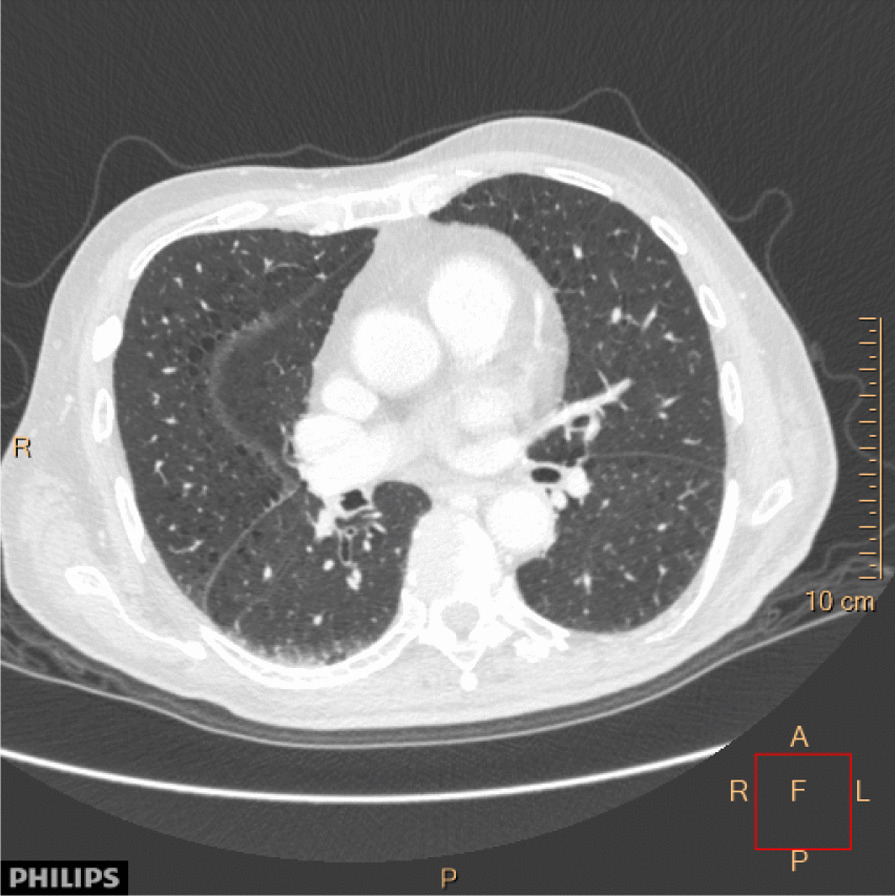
Computed tomography (CT) of the thorax axial view taken 2 months after the initiation of chemotherapy showing significant regression of the lesion in the lower lobe of the right lung. (A: anterior; R: right; L: left; P: posterior, F: focus; cm: centimeter)
